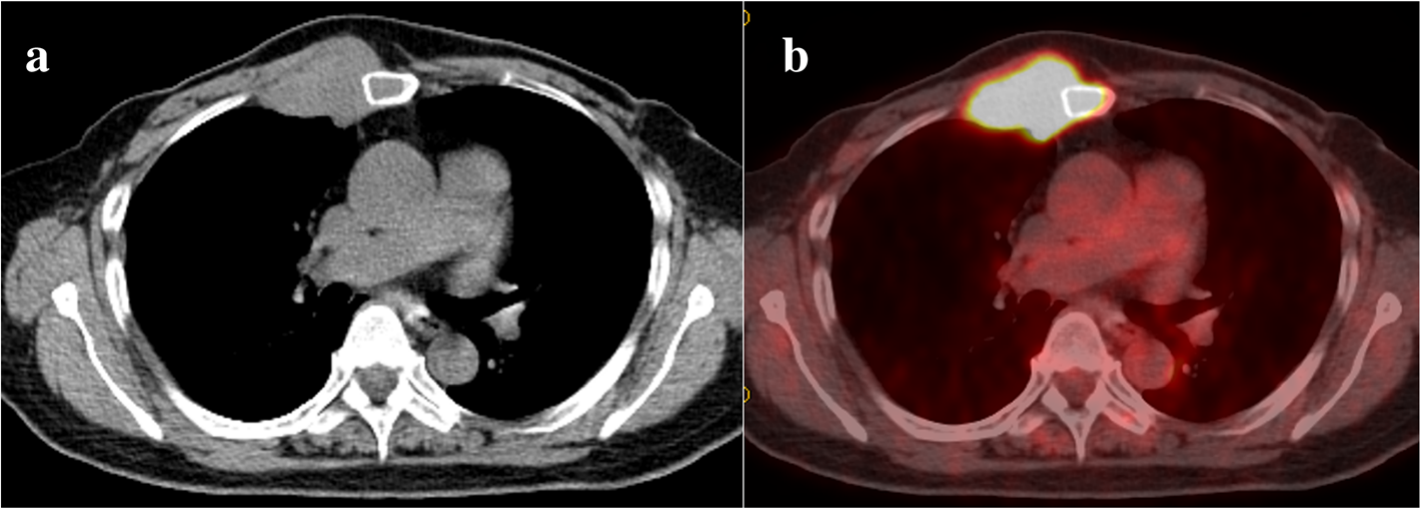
CT showing a 40 mm mass in sternum (a). PET-CT showing a standardized uptake value of [18f]-fluorodeoxyglucose of 7.30 on the mass in sternum (b)
